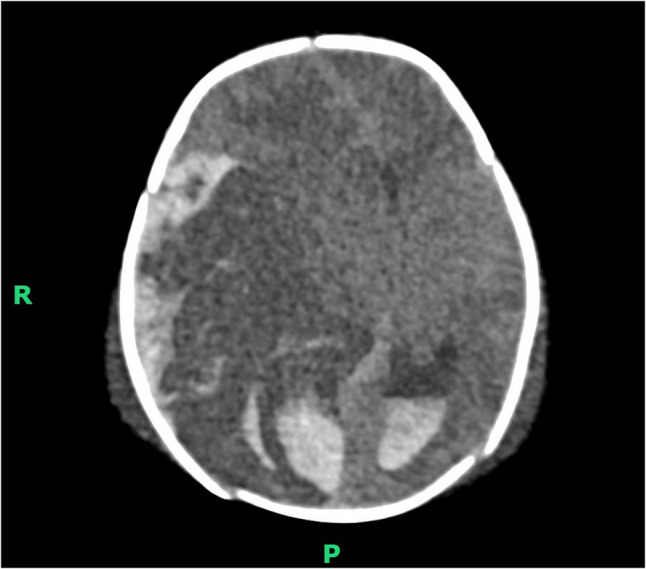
CT Head (axial view) demonstrating extensive haemorrhage and midline shift
